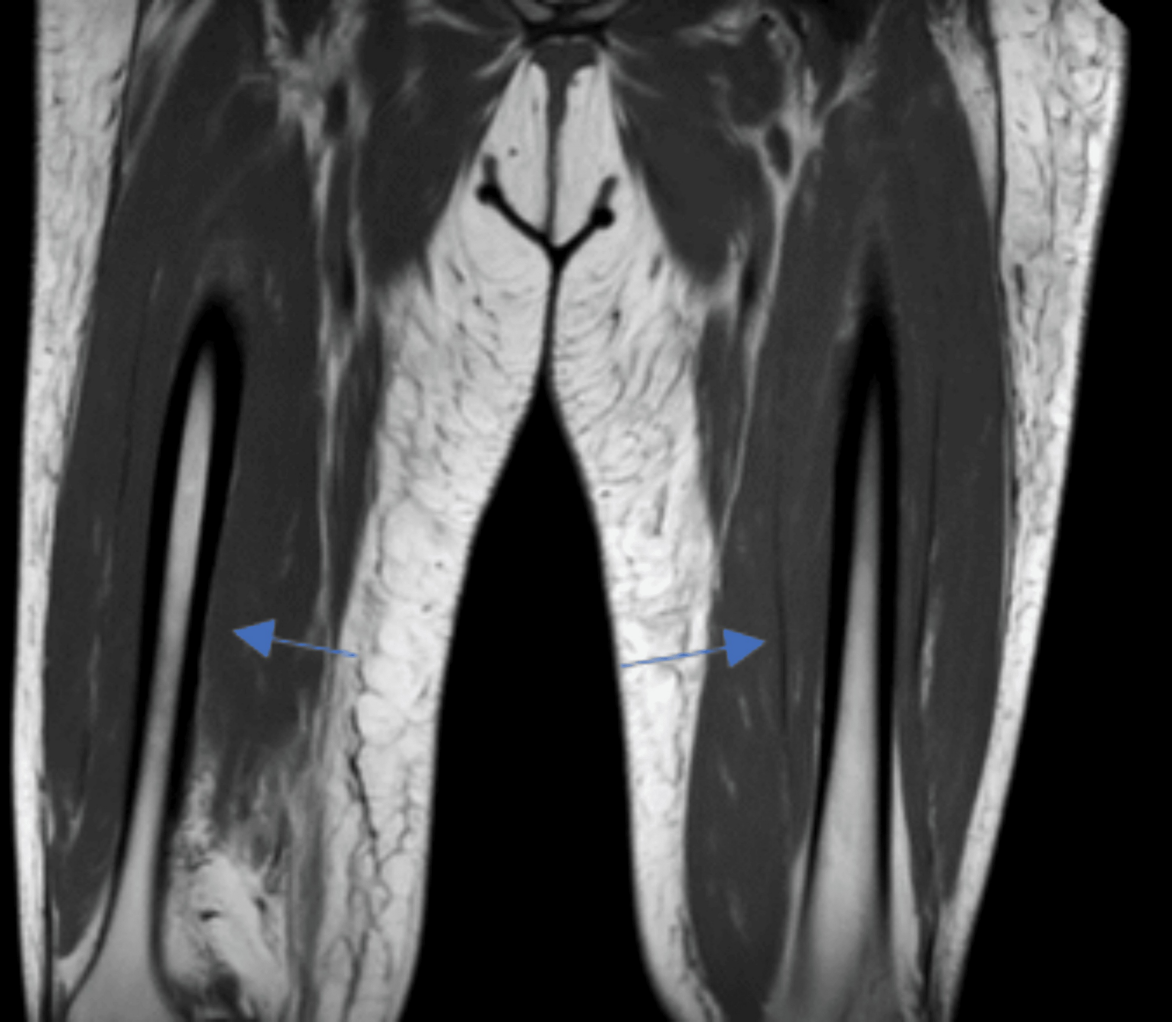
T1-weighted MRI of bilateral thighs showing the presence of edema
